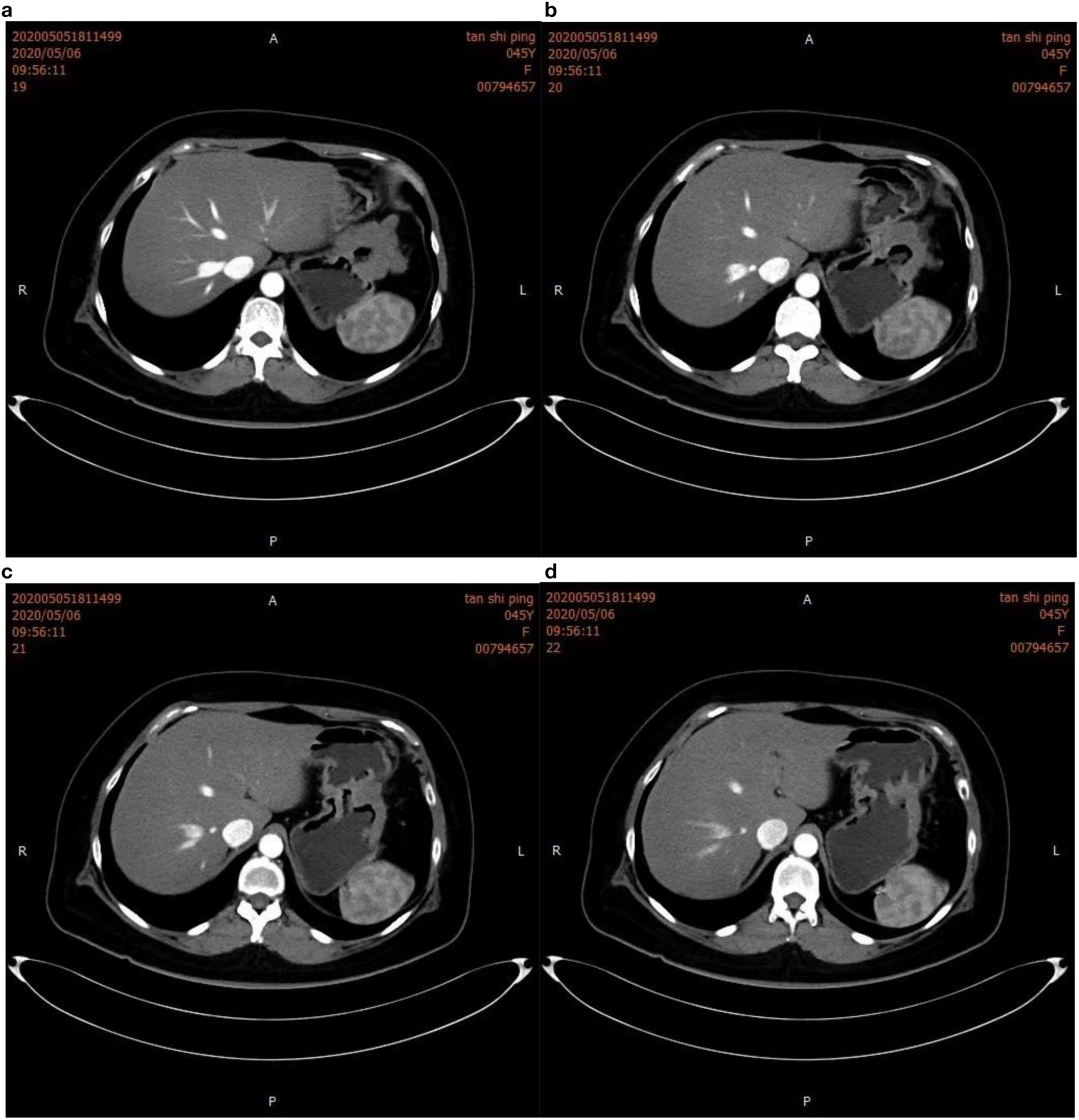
Intensified computed tomography (CT) scan of the abdomen. The image clearly shows gastric wall thickening in figure (a, b), characterized by uneven enhancement. Gastric lumen narrowing is also observed in figure (c, d), suggesting infiltrative growth of the tumor into the gastric cavity.
